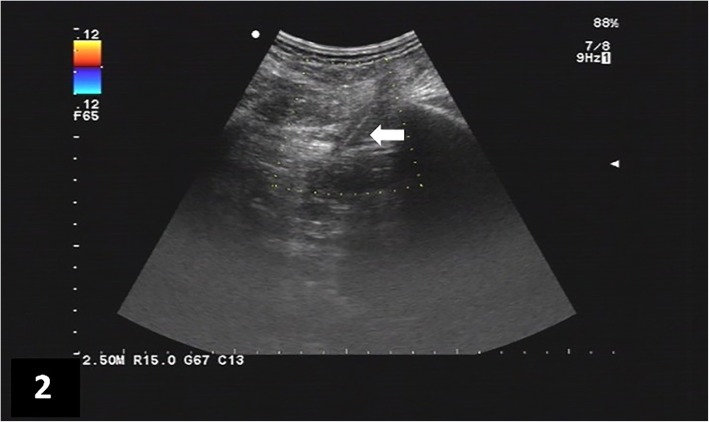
A hyperechoic linear lesion located in a low echo areal between the subcutaneous fat layer and intramuscular layer of the right flank was showed in color Doppler ultrasound image (arrow)
